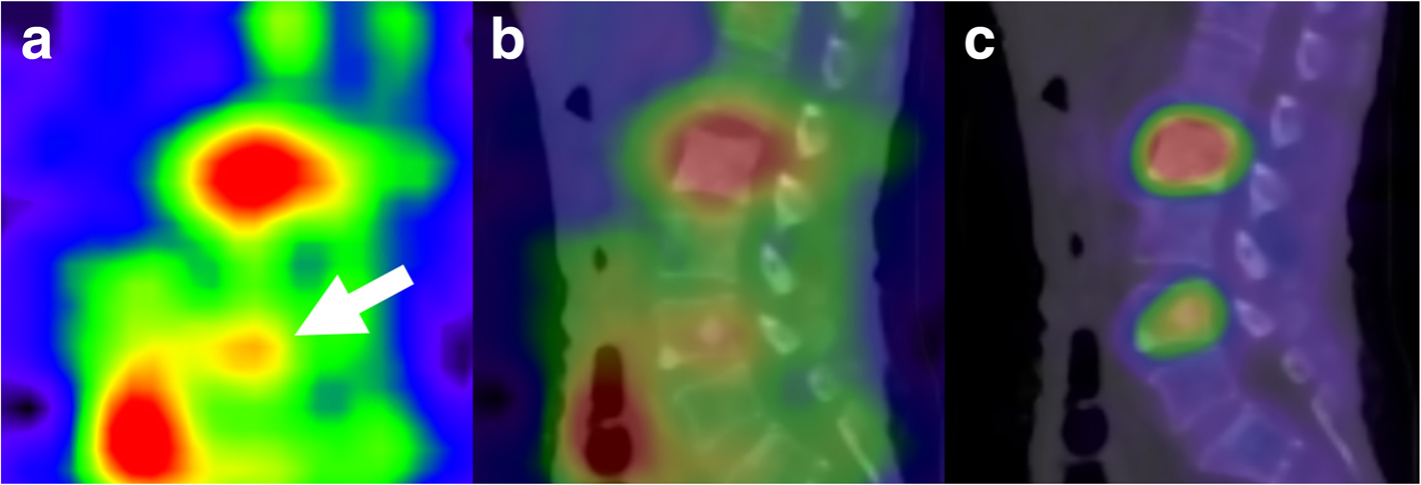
Coexistence of bowel uptake and bone metastasis in the lumbar spine revealed with Ra-223 SPECT (patient no. 6). SPECT (a) and SPECT/CT fused images (b) show that uptake in a bone metastasis at the fourth lumbar vertebra (arrow) is weak compared to adjacent bowel uptake. The corresponding Tc-99m HMDP SPECT/CT image (c) shows no bowel uptake. *HMDP* hydroxymethylene diphosphonate
